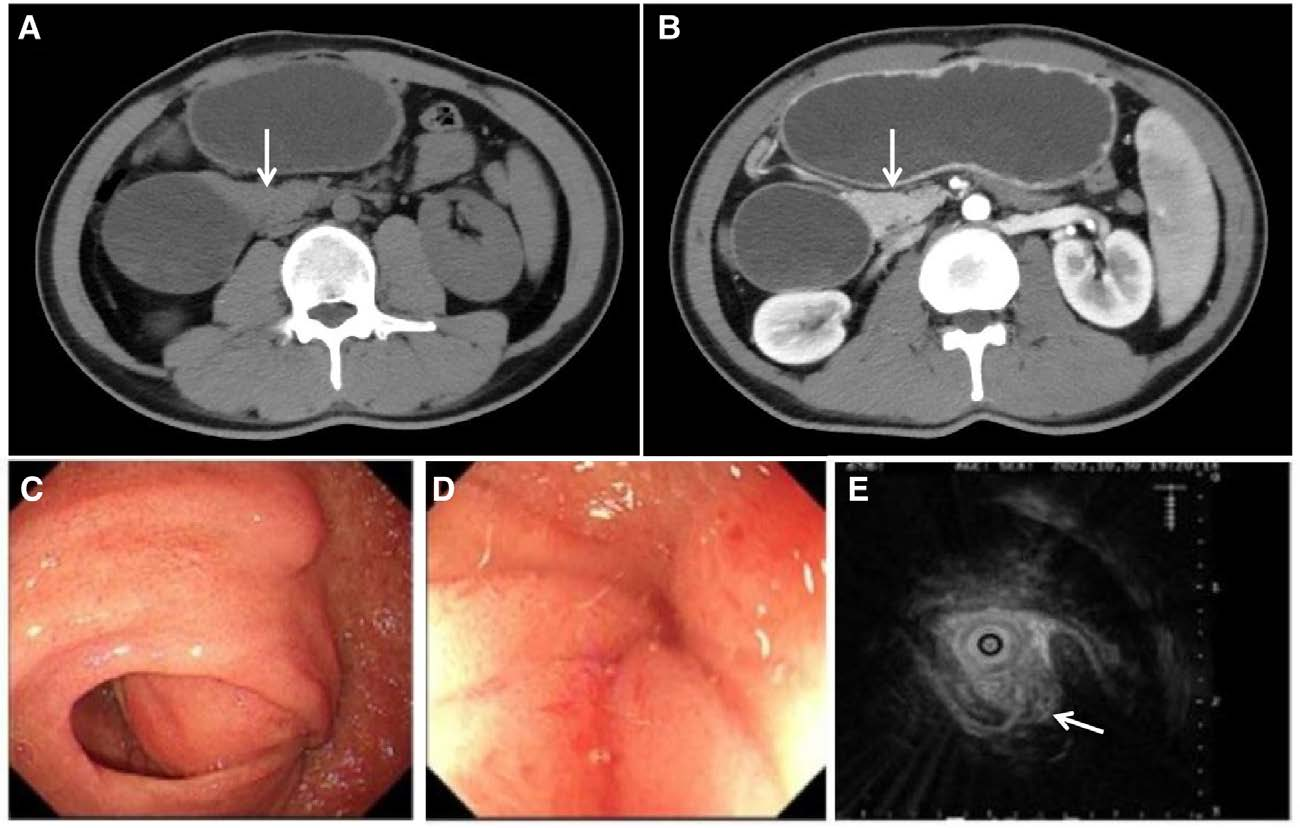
(A, B) CT with/without (i.v.) contrast (administration) revealed a large soft tissue mass in the descending part of duodenum, resulting in proximal intestinal dilation and gastric retention. (C) Gastroscopy showed a significant bulge in the descending segment of the duodenum, (D) narrowing the lumen and obstructing the passage of the endoscope. (E) Ultrasound shows a hypoechoic mass outside the descending duodenal wall, with no detectable boundaries.
